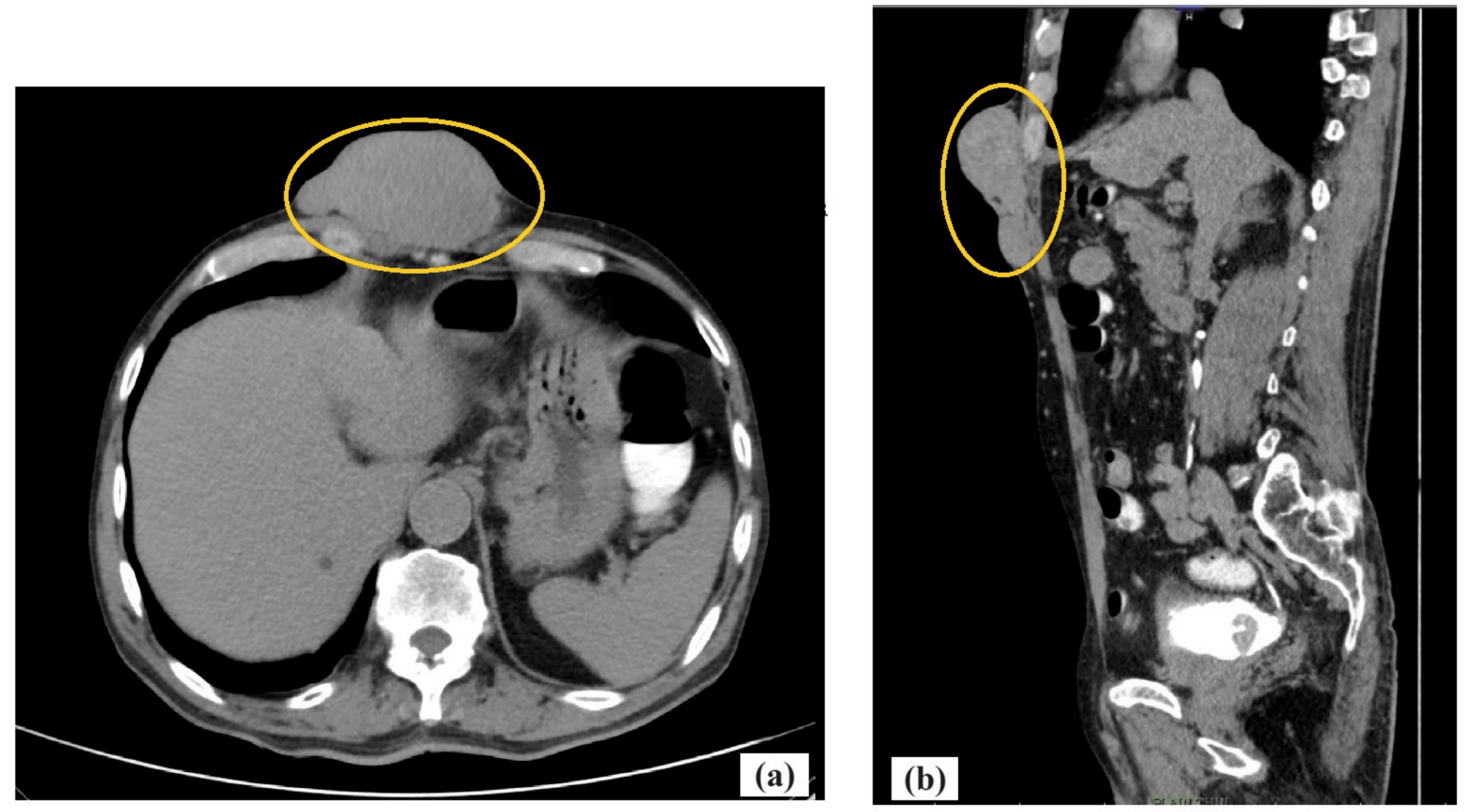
CECT abdomen and pelvis (a) Axial view showing lobulated soft tissue density mass lesion (yellow highlighted part) seen in subcutaneous plane in the anterior abdominal wall in the midline of epigastric region. (b) Sagittal view showing lobulated lesion (yellow highlighted part) with moderate heterogenous enhancement and loss of fat planes with underlying bilateral recti abdominis muscles. CECT - Contrast-enhanced computed tomography
